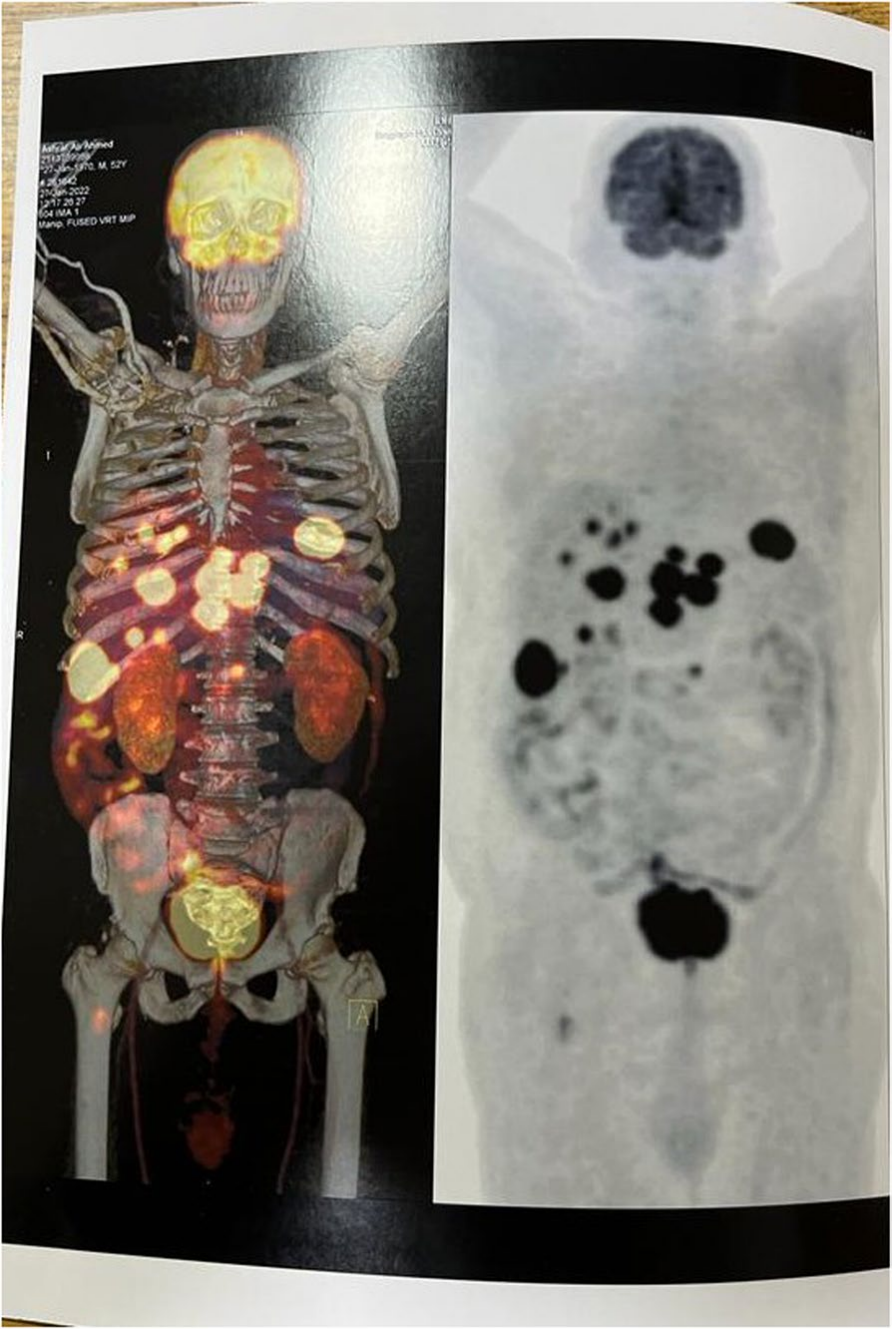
Whole-body PET-CT. Variable-sized metabolically active bilobar focal hepatic lesions in addition to active marrow-based osseous lesions at L1 vertebral body and proximal RT femoral shaft
